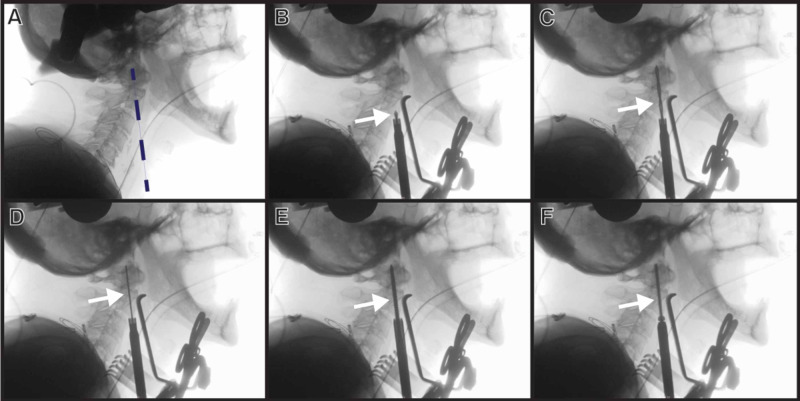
Planned trajectory of odontoid screw placement X-rays from the O-arm are used to (A) confirm planned trajectory (dotted line), (B) confirm drill position (drill tip indicated with the arrow), (C, D) visualize tapping (tap indicated with the arrow), and (E, F) visualize screw placement (screw indicated with the arrow). This eliminates the need for biplanar fluoroscopy and two C-arms
